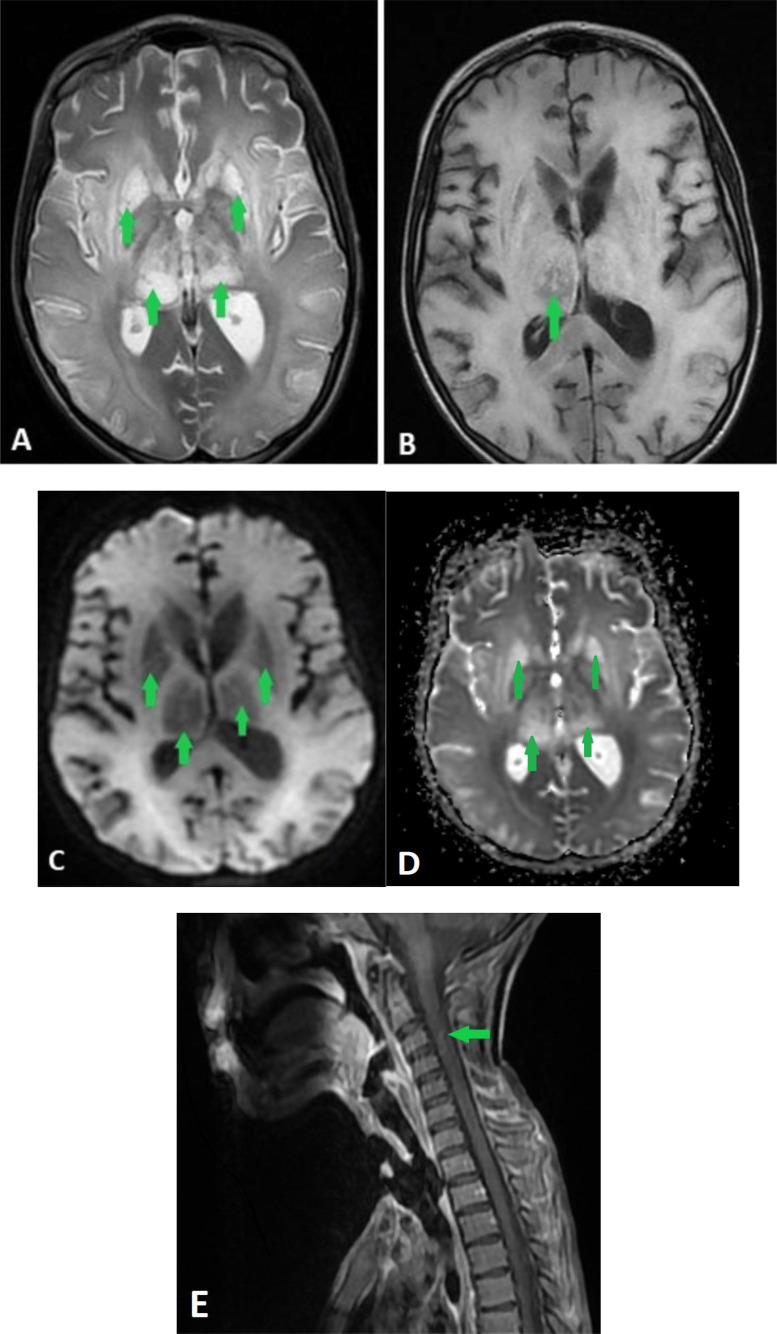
Control MRI after 1 month showing the vasogenic edema in the basal ganglia due to the late stage of the disease. A: T2 hypersignal of basal ganglia (green arrow), B: FLAIR hyposignal of right thalamus (green arrow), C: Diffusion hyposignal of basal ganglia (green arrow), D: facilitated ADC in basal ganglia E: T1 hyposignal of cervical spinal cord (green arrow).
